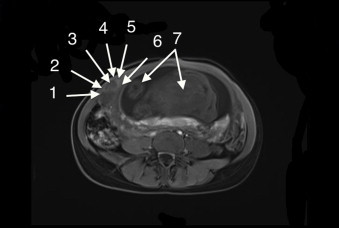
Abdominal Magnetic Resonance Imaging-T1 was performed and revealed uterine fibroid in Spigelian hernia (arrow). (1) Transverse abdominis. (2) Internal oblique. (3) Spigelian hernia. (4) Linea Semilunaris. (5) Rectus abdominis. (6) Uterus. (7) Fetus.
